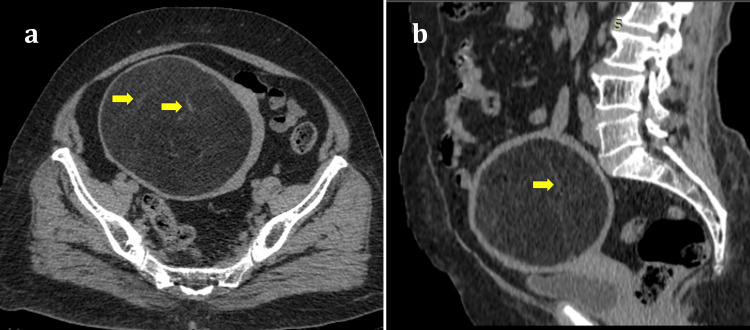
Plain CT features of uterine lipoleiomyoma Axial (a) and sagittal (b) non-contrast CT images of the pelvis demonstrating a large, well-circumscribed hypodense lesion arising from the uterus, showing near-homogeneous fatty attenuation with a few subtle internal soft tissue strands (arrows). CT: computed tomography
